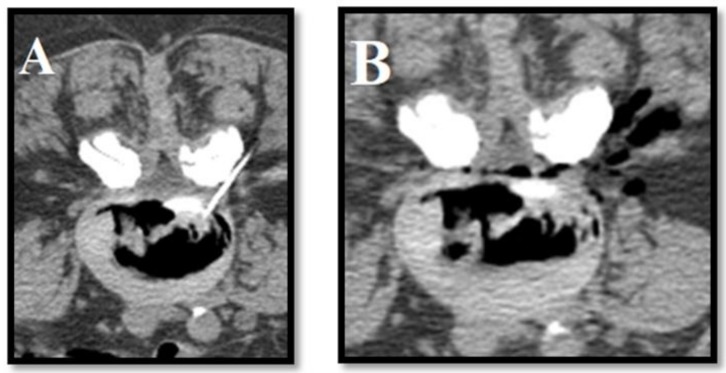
O_2_/O_3_: (A) intradiscal and (B) peri-radicular injection in patient with right lumbosciatalgia from L3-L4 herniated disk. Postero-lateral access is obtained via CT.
